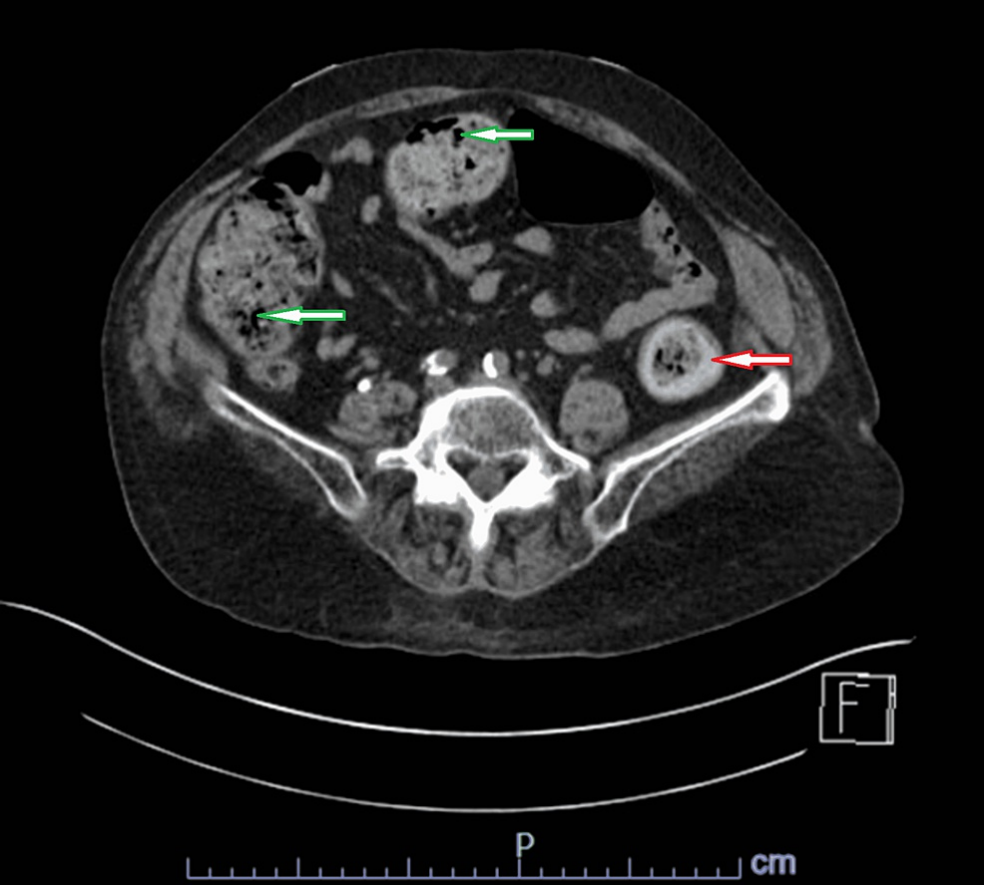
CT of the abdomen and pelvis without contrast showing a moderate amount of stool throughout the colon, and mural thickening in the rectum and proximal sigmoid colon. The green arrows indicate areas of moderate amount of stool in the colon. The red arrow indicates an area of mural thickening.
